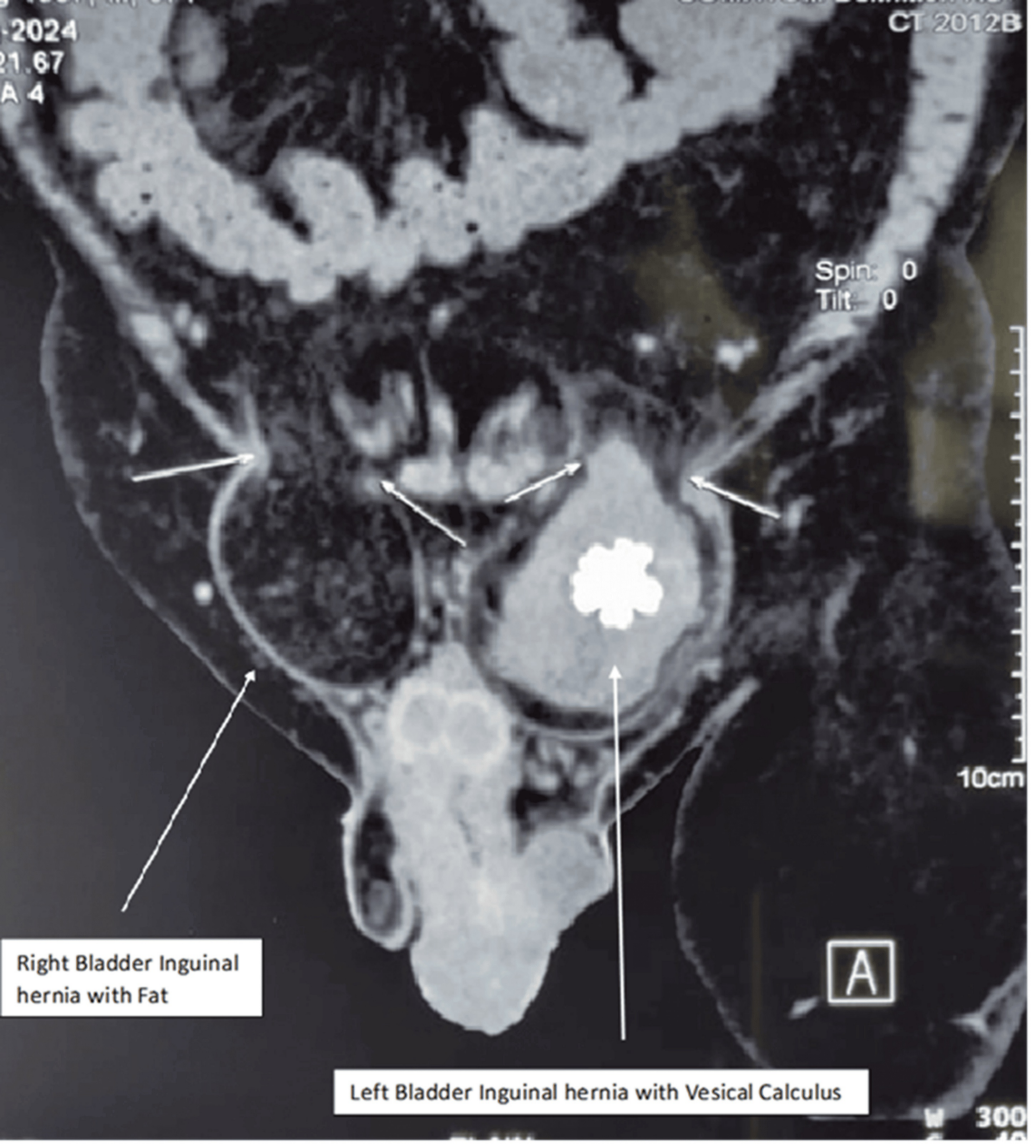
Computed tomography showing urinary bladder inguinal hernia with vesical calculus on the left side
